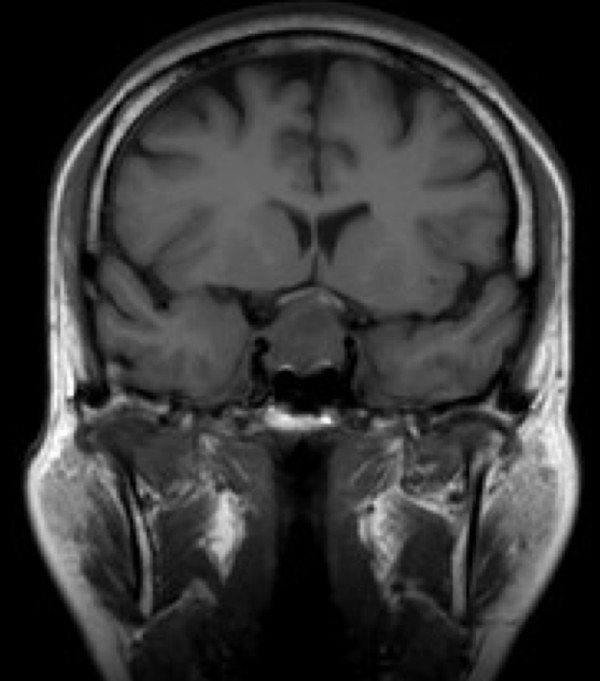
MRI scan demonstrating pituitary macroadenoma. A mixed cystic/solid mass is demonstrated lying within the pituitary fossa, bowing and indenting the optic chiasm.
